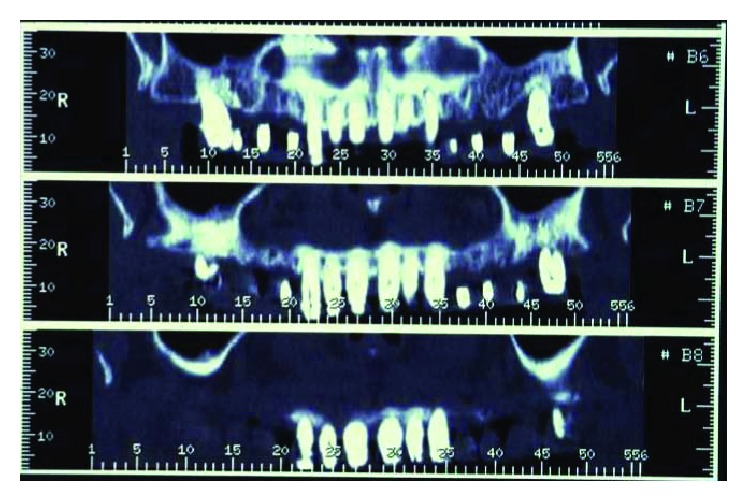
CT scan for evaluation of implant sites.
